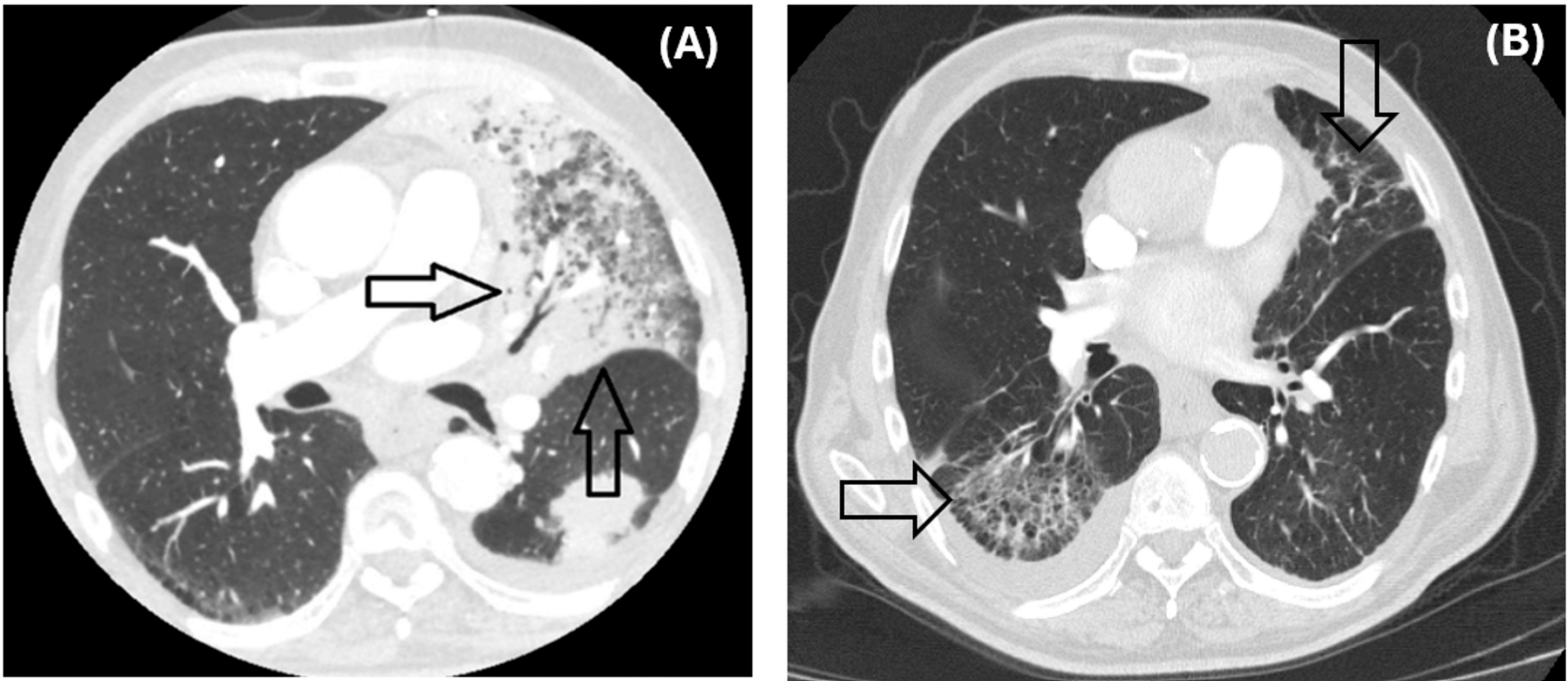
Pneumonia G2 and pneumonitis G1 (A) After three cycles of treatment, CT scan revealed left upper lobe consolidative pneumonia. The patient had shortness of breath and decreased SpO₂ (87%). Diagnosis: pneumonia grade 2. (B) After hospitalization and treatment with antibiotics, the consolidative pneumonia resolved. CT scan showed pneumonitis. The patient was asymptomatic at that time. Diagnosis: immunotherapy-induced pneumonitis grade 1.
